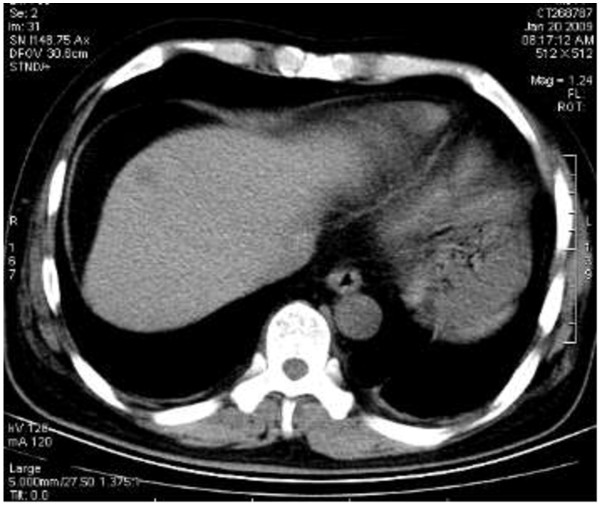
Low density nodule with hazy border close to the liver capsule in the front part of left liver shown by a plain CT scan.
